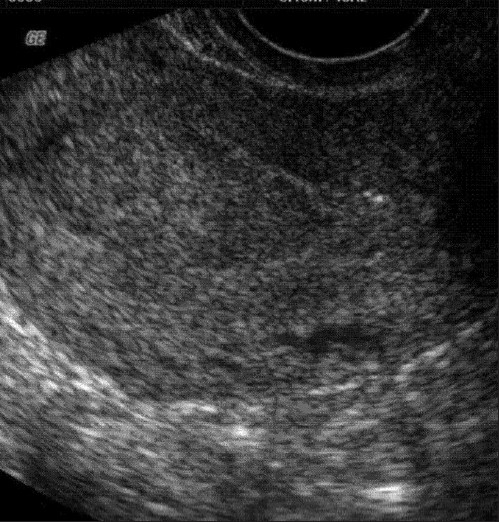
Ultrasound showing thickened endometrium
